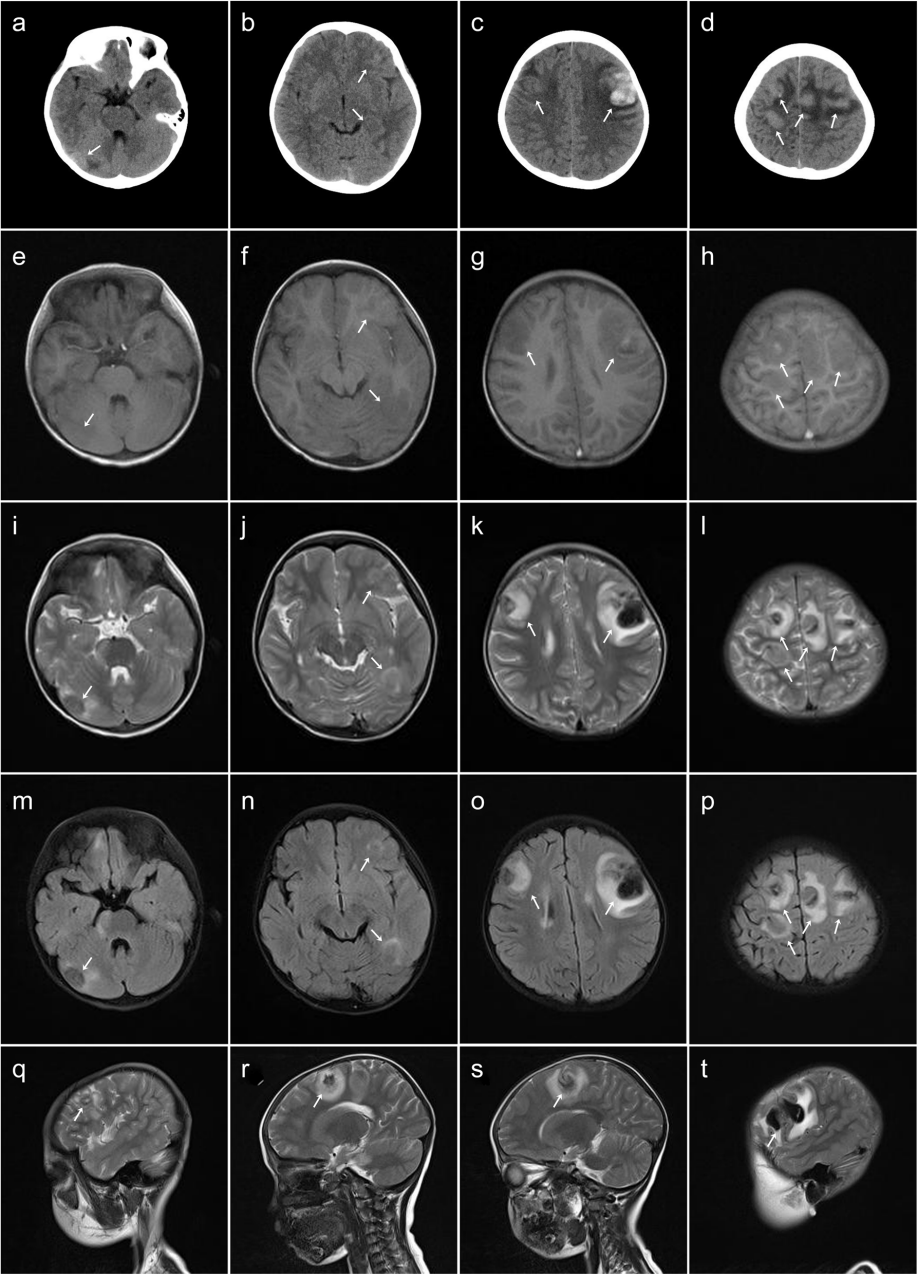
Brain MRI and CT images for the case 1. a–d Axial CT image showing lesions in the right cerebellar hemisphere, left occipital lobe, pons and frontal-parietal lobes with slight hyperdensity (white arrows), patchy hypodense edema, and hyperdensity. e–h Axial T1-weighted imaging showing hypointense and heterogeneous hypo- and hyperintense lesions (white arrows) with high signal indicating hemorrhage. i–l Axial T2-weighted imaging showing extensive peri-lesional edema (white arrows). m–p Axial T2-fluid-attenuated inversion recovery images showing extensive peri-lesional edema (white arrows). q–t Sagittal T2-weighted image showing extensive peri-lesional edema (white arrows)
